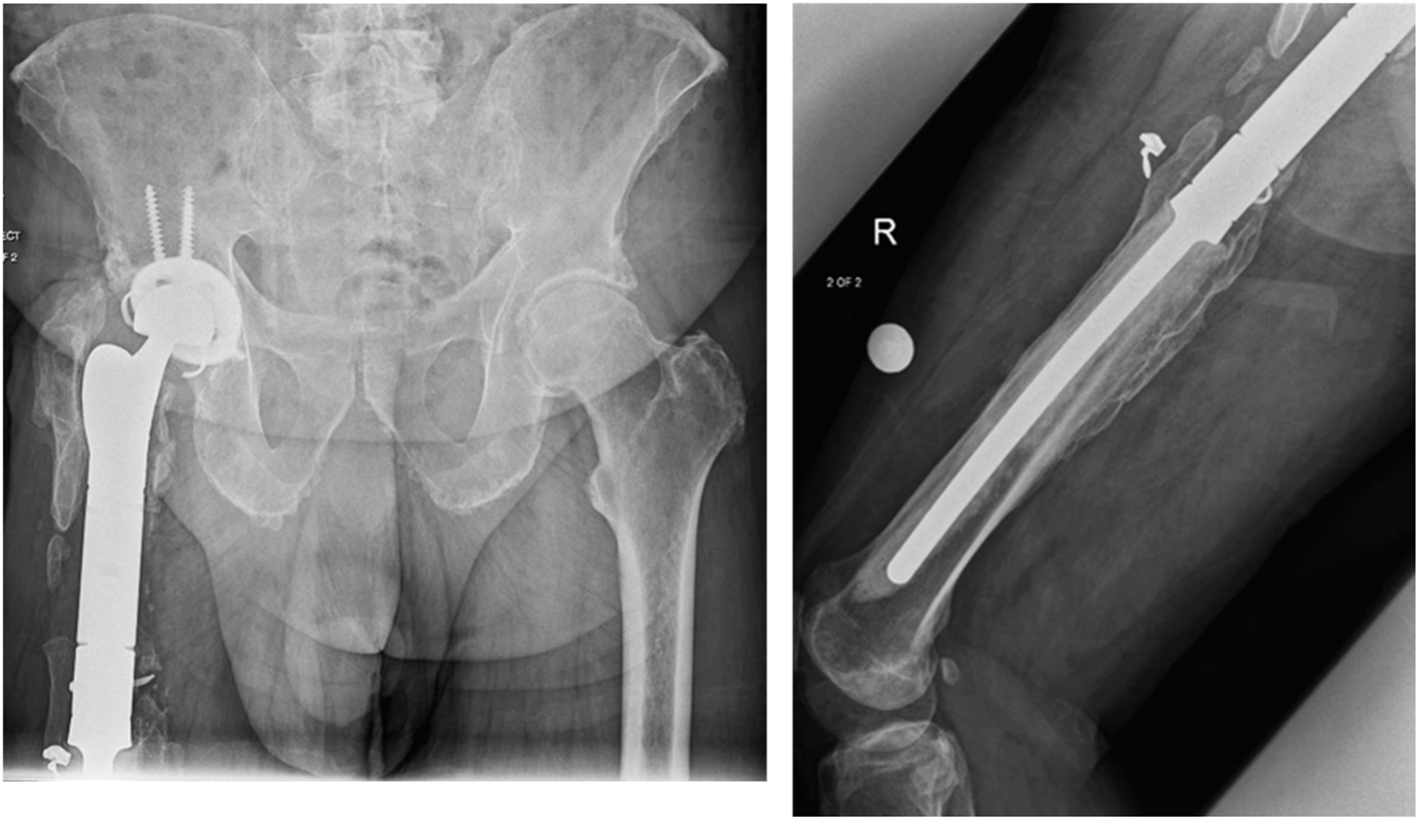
Corresponding radiographs taken at 132 month follow-up, post-DAIR surgery
